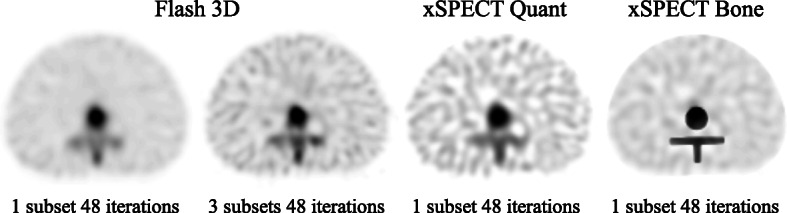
Representative transaxial images of SPECT datasets including three reconstructions at TBR4. Reconstructed images showed the xSPECT and F3D images with 1 subset and with 3 subsets at 48 iterations, respectively
